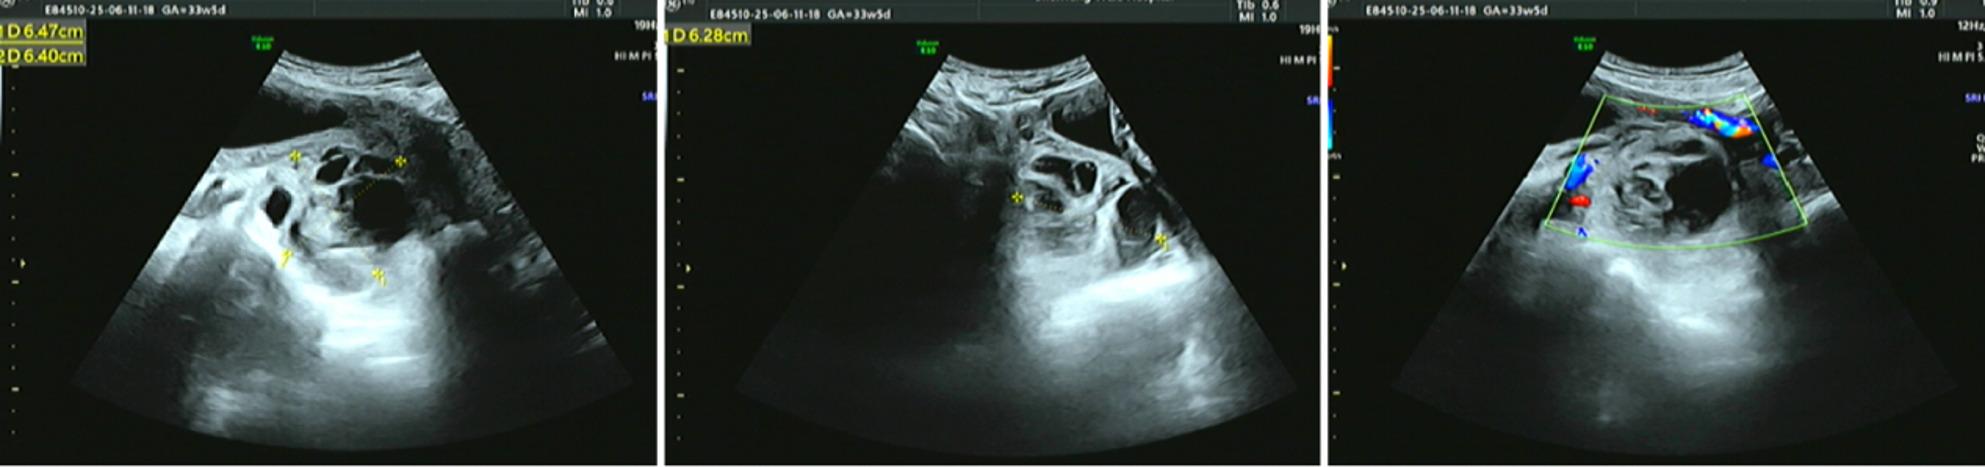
Follow-up transvaginal ultrasound on the first postoperative day. The cyst measures 65×63×63mm with no signs of active bleeding
